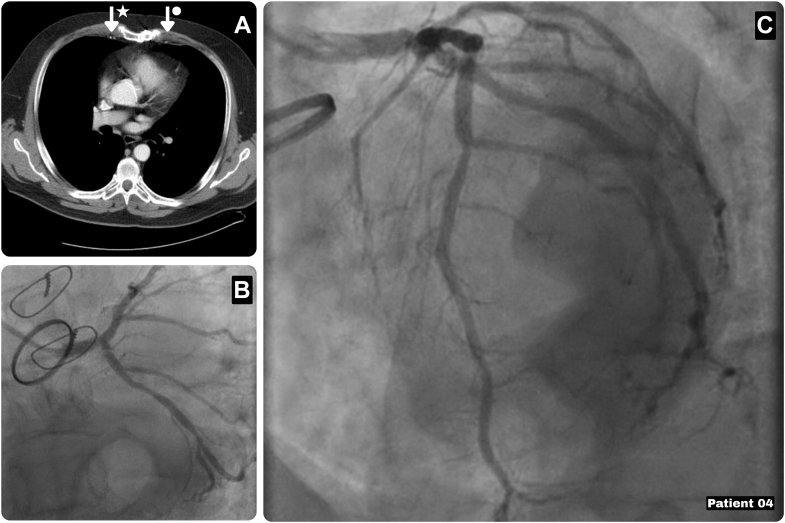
Characteristics of Patient 4 (A) Preoperative computed tomography showing the LIMA (arrow with dot) and RIMA (arrow with star). (B and C) Preoperative coronary angiography with a mechanical aortic valve in situ and a calcified left main coronary artery and a functionally significant LAD lesion (fractional flow reserve: 0.72). LAD = left anterior descending artery; LIMA = left internal mammary artery; RIMA = right internal mammary artery.
